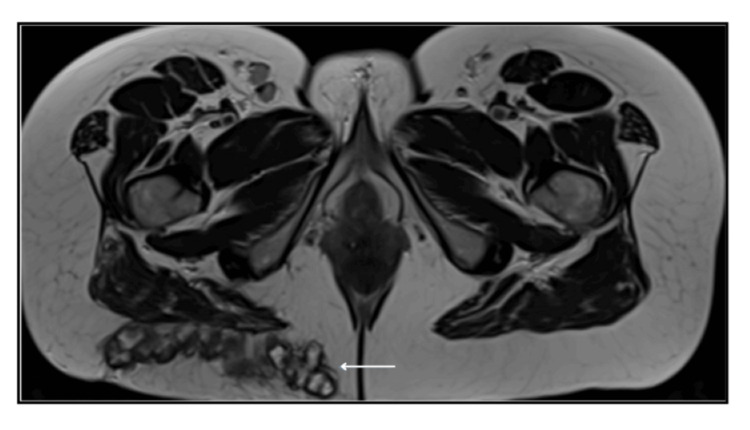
Axial T2W large field of view MRI image of the pelvis post embolisation. Infarction of subcutaneous fat within the right buttock with thick-walled encapsulated fat lobules (arrow)
